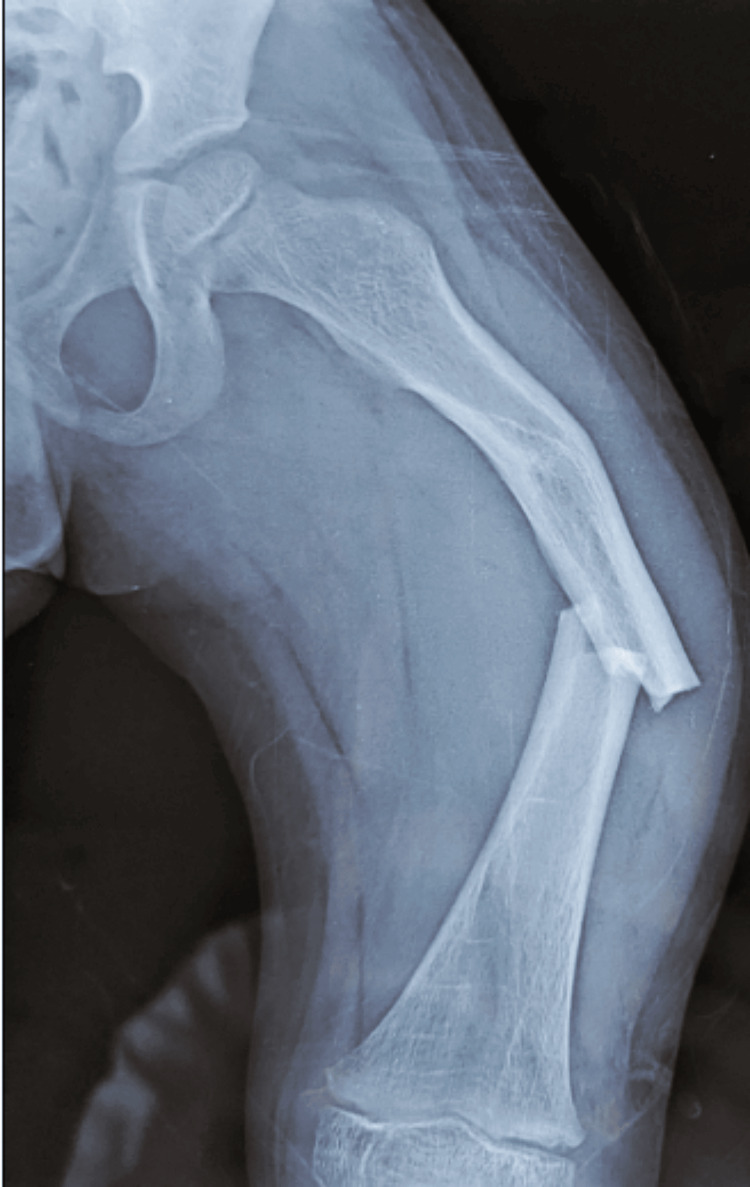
Anterior radiograph of the femur in a child with OI showing a displaced transverse fracture This image highlights the bone fragility characteristic of OI, with the fracture occurring despite minimal trauma. OI: osteogenesis imperfecta
